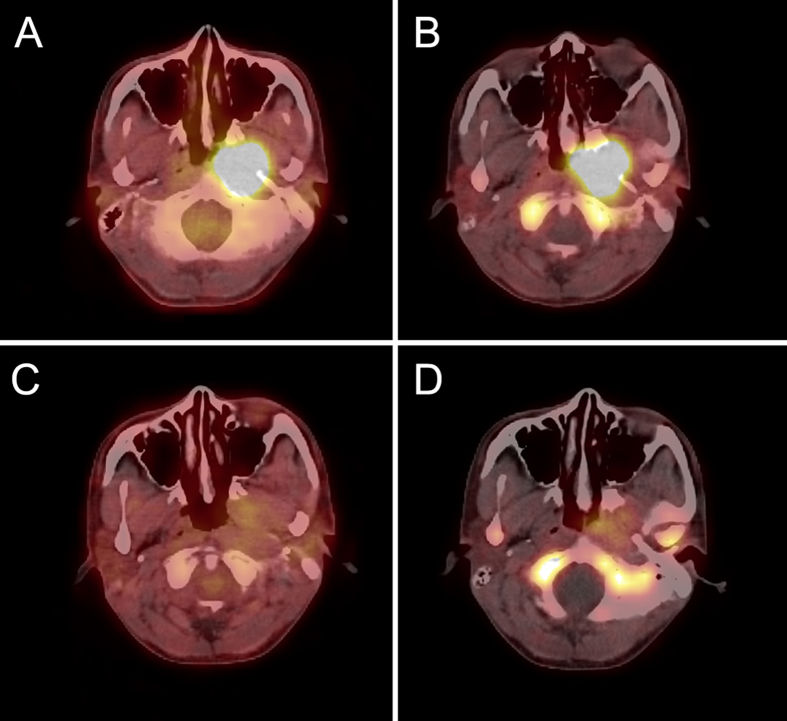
A 22-year-old male NPC patient, pre-treatment PET/CT showed high FDG (A) and FLT (B) uptake in primary lesion, SUVmax = 9.5 and 8.9, respectively. However, after two cycles of NACT, only mild FDG (C) and FLT (D) uptake was detected, SUVmax = 2.1 and 2.9. At the end of radiotherapy, it was diagnosed CR.
